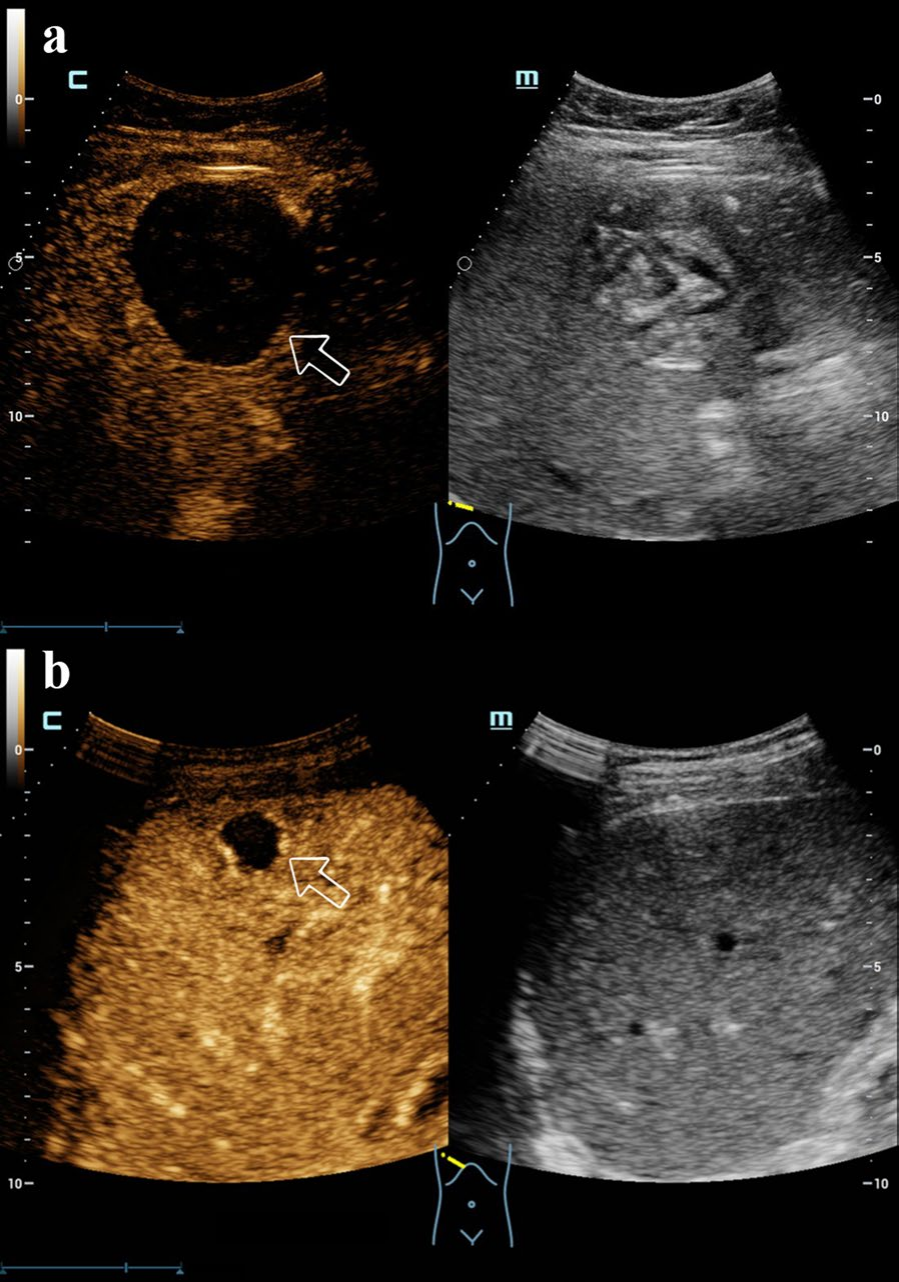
The CEUS ultrasonogram of CE and AE. a A CE lesion showed enhancing circumscribed boundary and non-enhancement in the arterial phase (white arrow), indicating that the lesion may not invade the parenchyma of the surrounding normal liver. b An AE lesion showed enhancing ill-defined boundary and hyper-enhancement in the arterial phase, whose enhancement pattern was the circular ring of enhancement with non-enhancement areas within the lesion (white arrow), suggesting that there may be inflammatory reaction zones around the lesion and the lesion may contain marginal microvessels with more abundant blood supply. *CEUS* contrast-enhanced ultrasound, *CE* cystic echinococcosis, *AE* alveolar echinococcosis
